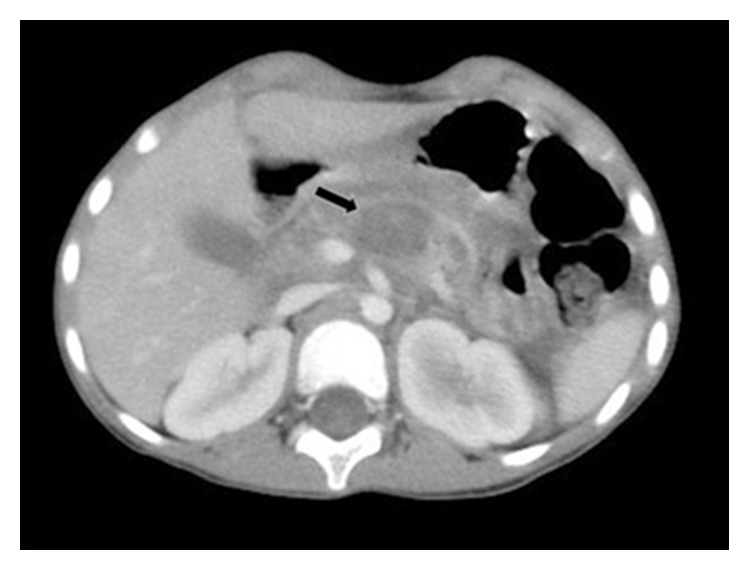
Abdominal enhanced CT showing a cystic low-density shadow (arrow) in the head and neck of the pancreas.
